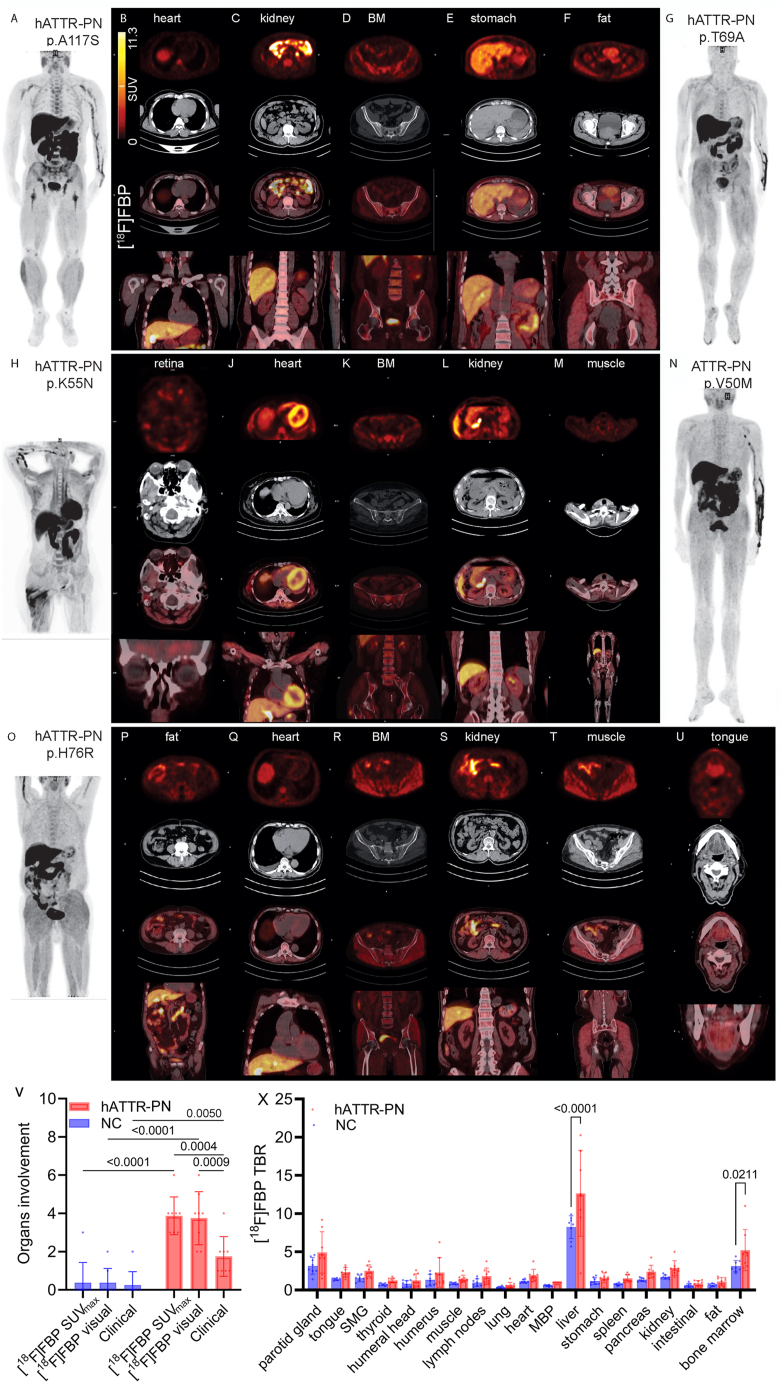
[^18^F]FBP imaging in hATTR-PN patients and normal controls for assessing organ involvement. (A, G, H, N, O) Maximum intensity projection (MIP) of [^18^F]FBP whole-body scans of hATTR-PN patients. Transaxial PET, CT, transaxial and coronal images of overlay images of the heart (B), kidney (C), and bone marrow (D) from a patient with hATTR-PN (p.A117S, aged 48 years old, male, A); stomach (E), and fat in the pelvic floor (F) from a patient with hATTR-PN (p.T69A, aged 45 years old, female, G); retina (I), heart (J), and BM (K) from a patient with hATTR-PN (p.K55aN, aged 60 years old, female, H); and kidney (L) and muscle (M) from a hATTR-PN patient (p.V50M, aged 67 years old, male, N). and fat (P), heart (Q), kidney (R), muscle (S), and tongue (U) of a hATTR-PN patient (p.H76R, aged 82 years old, male, O). (V) Comparison between clinical assessment and visual and SUV_max_ analysis of [^18^F]FBP for organ involvement in NC (normal control) and hATTR-PN patients. (X) Regional uptake of [^18^F]FBP in NC and hATTR-PN patients by target-to-background ratio (TBR) analysis. hATTR, hereditary transthyretin amyloidosis; PN, polyneuropathy; MBP, Mediastinal blood pool; SMG, submandibular gland.
